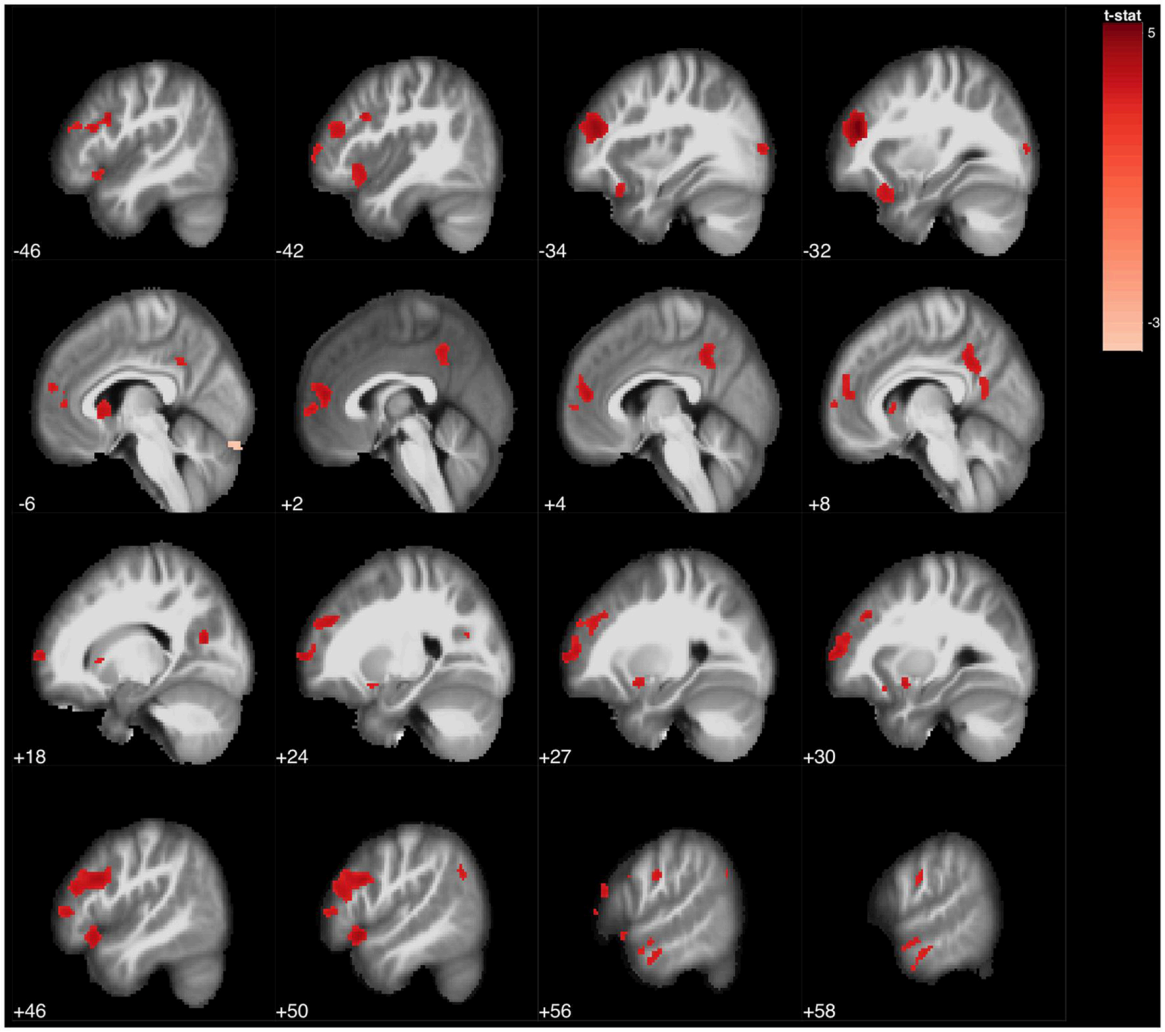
Statistical parametric map overlaid on an MRI template (neurological orientation, sagittal view), showing brain regions positively correlated with Tower of London (uncorrected *p* < 0.001).
